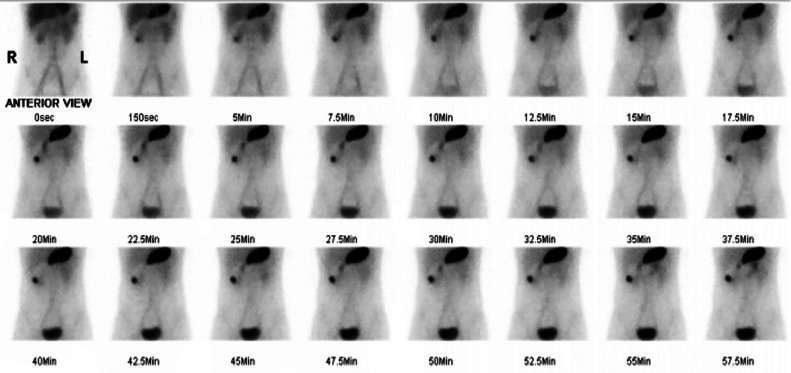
Tc-99m pertechnetate scintigraphy or Meckel's scan identified a focal uptake within the right upper hemiabdomen which coincides with normal radiotracer uptake in the stomach in sequential anterior planar images acquired over 60 minutes (2.5 min/frame).
